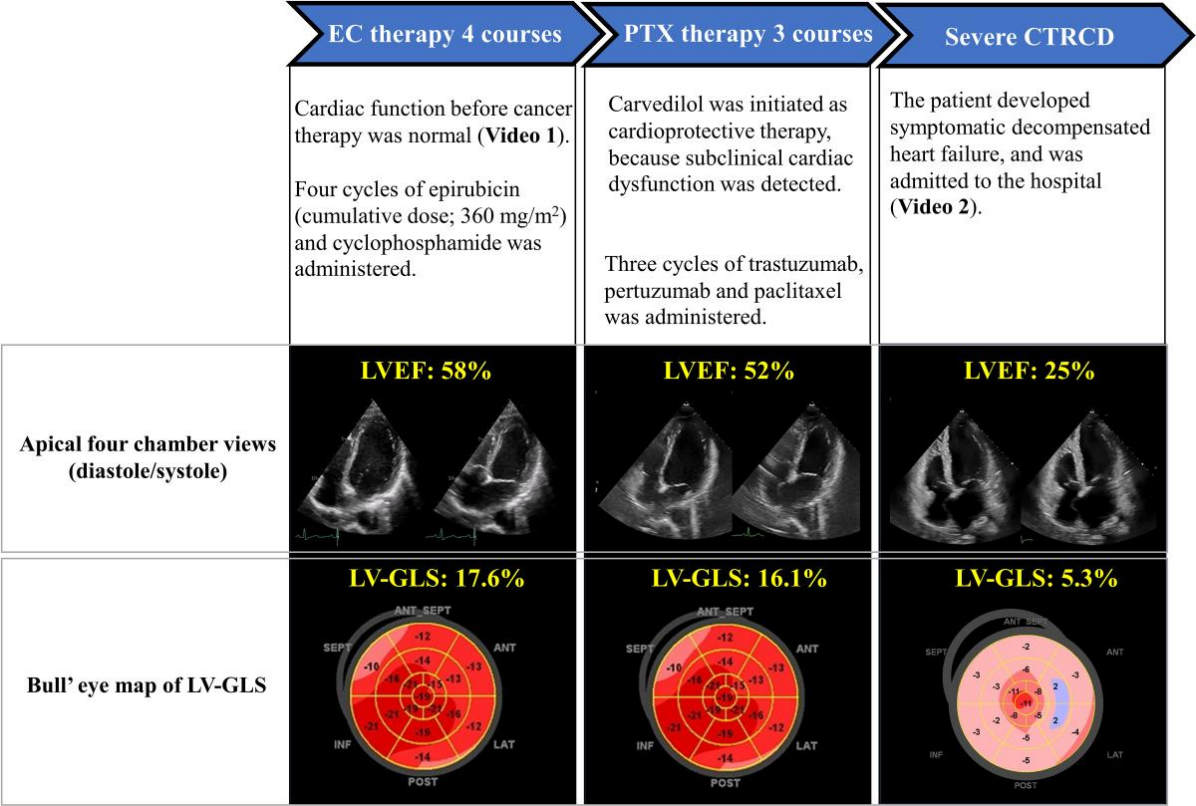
Timeline of the clinical course before admission. Cardiac function was normal prior to the initiation of cancer therapy. The patient received four cycles of epirubicin (cumulative dose, 360 mg/m^2^) and cyclophosphamide. Carvedilol was initiated as cardioprotective therapy because subclinical cardiac dysfunction was detected on echocardiography. After three cycles of pertuzumab, trastuzumab, and paclitaxel therapy, the patient developed symptomatic decompensated heart failure and was admitted to the hospital. LVEF, left ventricular ejection fraction; GLS, global longitudinal strain.
